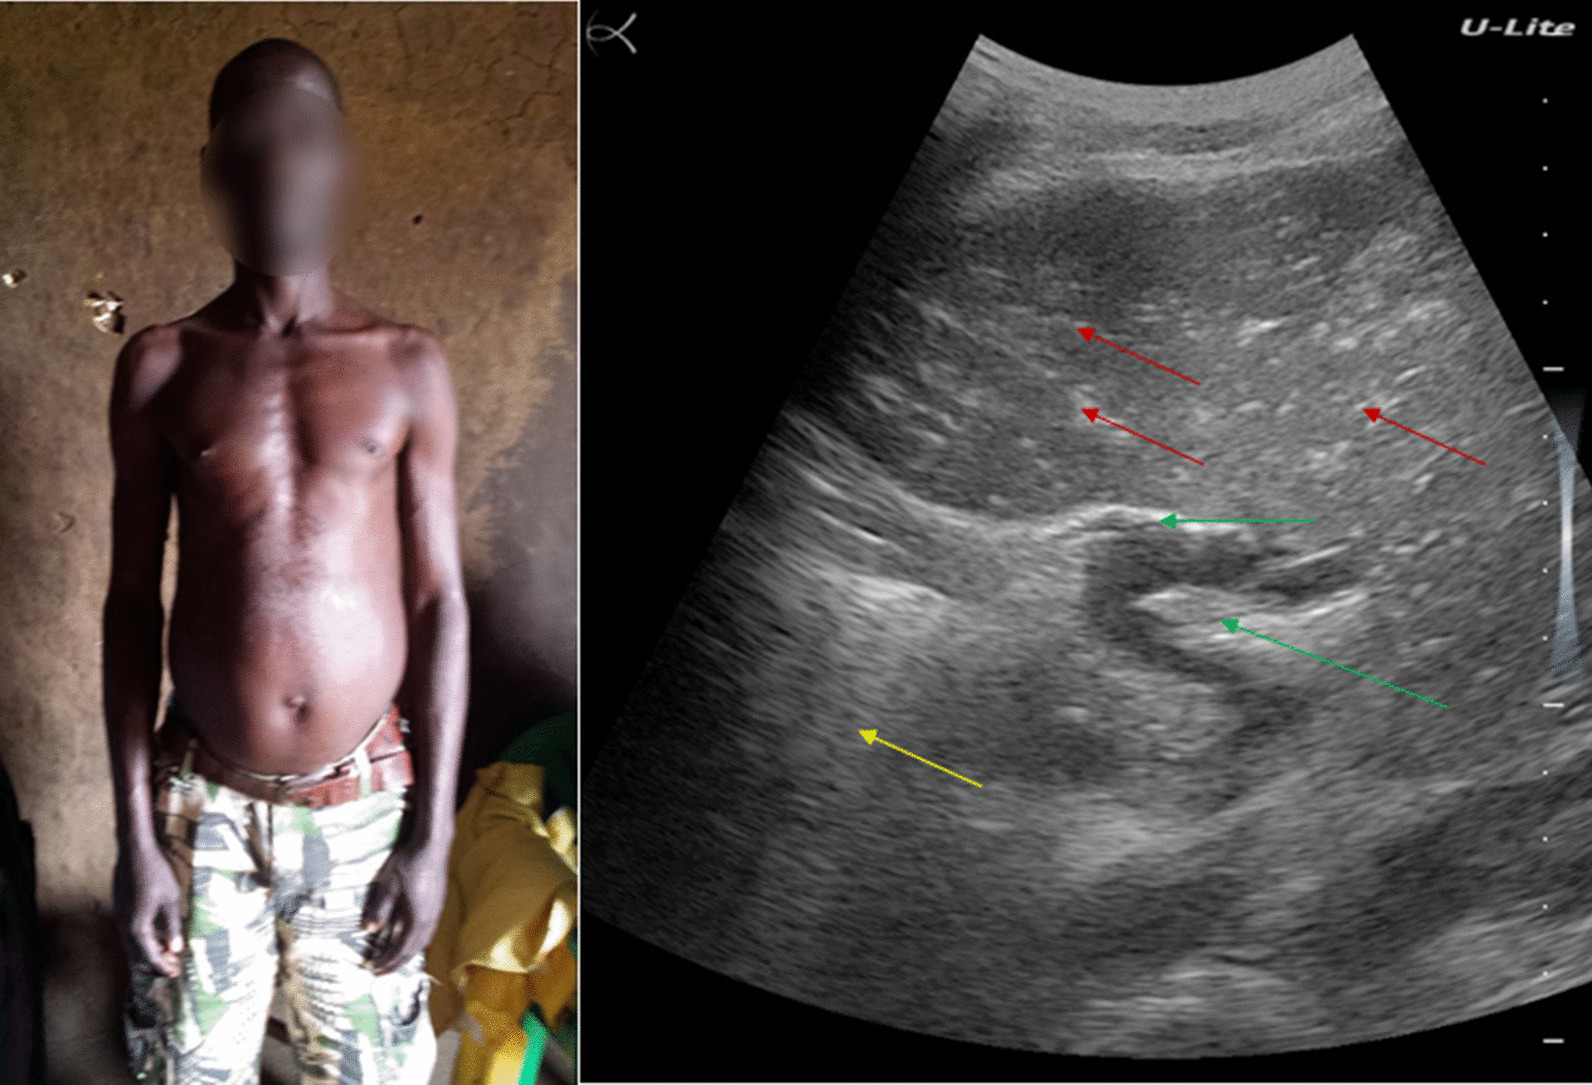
Patient #4 (left). Ultrasound examination (right) showing distorted liver architecture and heterogeneous echotexture of liver parenchyma and starry night sky liver pattern (red arrow), decreased definition of the portal vein wall (green arrow), and posterior reinforcement (yellow arrow)
